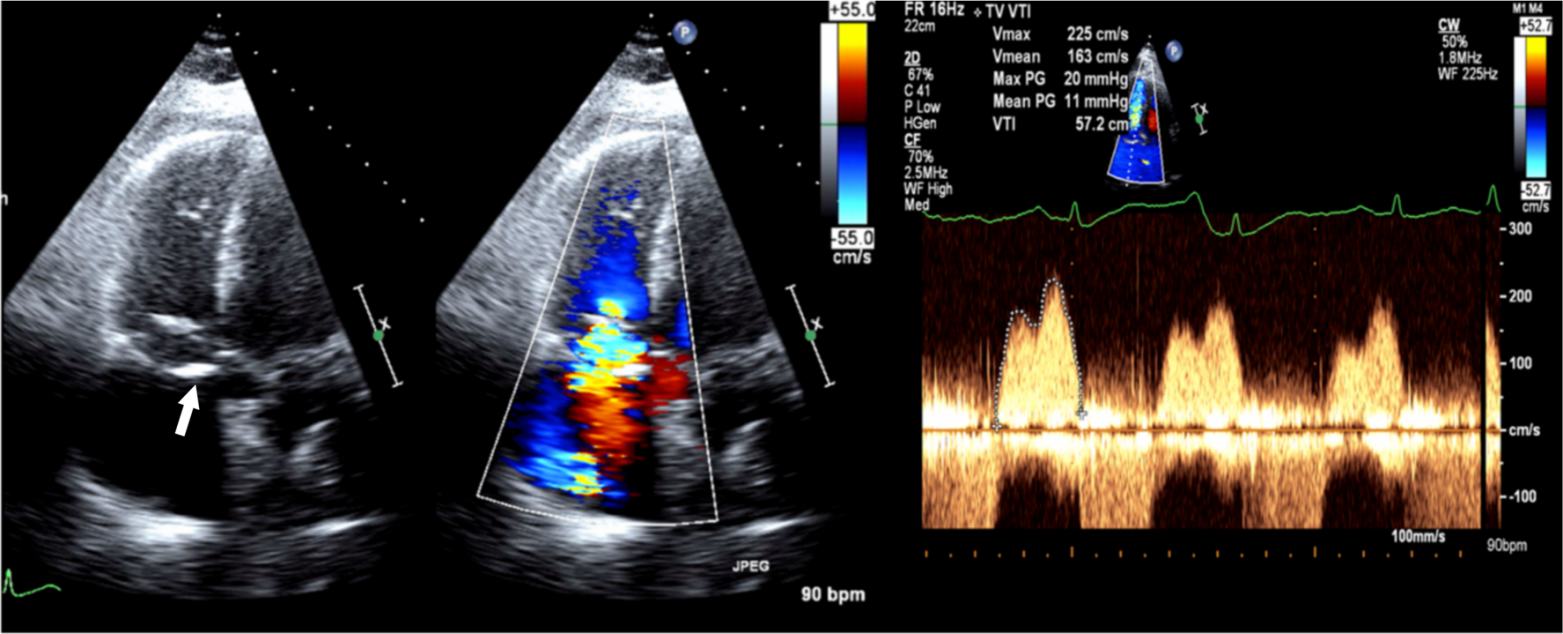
Tricuspid stenosis in echocardiogram. 2D and color Doppler images in a patient with anti-phospholipid antibody syndrome shows thickening of the valve leaflets (*arrow*) and continuous-wave Doppler with a mean pressure gradient of 11 mmHg, which is consistent with severe tricuspid stenosis
